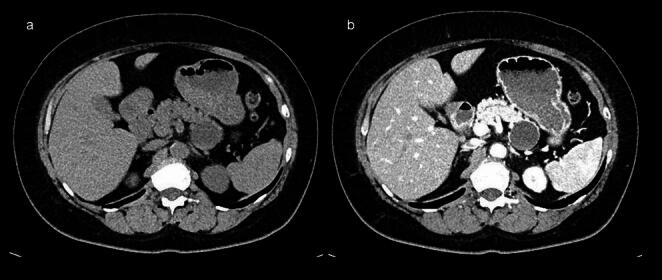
Abdominal computed tomography (CT). a non-enhanced CT. b Early phase of arteries on contrast-enhanced CT. A large, unilocular, 35-mm cystic mass was found in the pancreatic tail. Non-contrast CT showed mild hyperintensity within the mass
